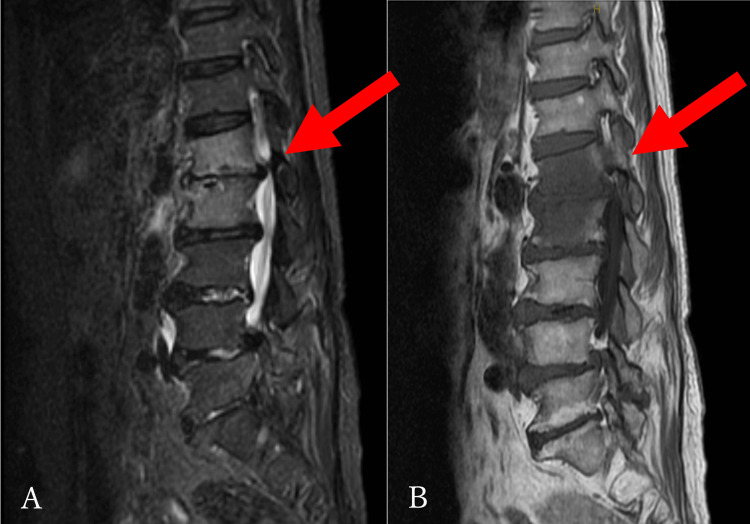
MRI findings of the entire spine. A, B: Short inversion time inversion recovery revealing a new high intensity at the L1 and L2 vertebral bodies, with T1 revealing a low intensity (arrow).
